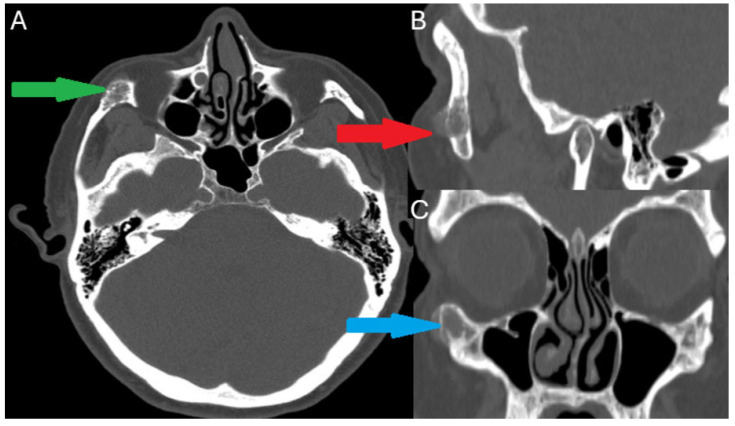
Because of atypical bone symptoms, more improved diagnostics were performed. Initial CT scan with contrast enhancement (GE Medical Systems, Optima CT 540, Bengaluru, India). In the axial view (green arrow), a radiolucent lesion of low density is seen within the body of the right zygomatic bone. The lesion has dimensions of 1.5 × 1.0 cm and a trabecular appearance (A). Within the lesion, radiopaque spikes are seen radiating outwards of the lesion following a sunburst pattern. On the lateral view, the red arrow points out that the lesion is well circumscribed, although the cortical layer of the zygomatic bone is eroded (B). There are no signs of periosteal reaction. Some atypical bone swelling and elevation with a mixed radiolucent-radiopaque lesion on the border of the inferior orbital rim and the zygomatico-maxillary junction is noted (blue arrow) on the coronal view (C). Similar radiologic findings in this area should be differentiated from fibrous dysplasia, osteochondroma, meningioma, osteogenic sarcoma, osteomyelitis, atypical bone inflammation, and multiple myeloma (MM). Based on the radiological findings, the suspicion of a benign intraosseous lesion was high. Because of the atypical location, symptoms, and radiological appearance, a decision for an excisional biopsy was made. We avoided the performance of a fine needle aspiration biopsy (FNAB), since the bone that covers the lesion makes it hard to penetrate, and the possibility of bleeding was high [1]. This decision was made because there were no worrisome symptoms like fatigue, weight loss, or anemia. On the other hand, because of the radiological appearance, authors initially differentiated the lesion between ossifying fibroma (OsF), CGCG (central giant cell granuloma), or an intraosseous hemangioma (IH). The suspicion for IH was high because of the characteristic pattern in CT scans. A well-circumscribed lesion with clear borders and a radiating trabecular appearance following a ‘’sunburst ‘’pattern can be pathognomonic for IH [2]. MRI can add valuable information regarding soft tissue involvement and is superior to a CT scan for the evaluation of vascular lesions [3]. Some studies reported that on MRI, IH are typically seen as hyperintense lesions on T2 images and hypointense or hyperintense on T1 images depending on the size of the lesion [3,4]. Generally, in radiological examination, after contrast, the lesion is more visible; however, small lesions are seen as hyperintense while larger lesions are hypointense on T1-weighted images [3,4]. The ratio of red and yellow (fatty) marrow within the lesion influences the MRI appearance of IH [2]. It is always worth estimating the periosteal condition and the status of adjacent bones. The absence of periosteal reaction made osteogenic sarcoma a less possible outcome than other entities. Intraosseous meningioma was also excluded because of the atypical site of that lesion and the absence of soft tissues. Fibrous dysplasia has a ground-glass appearance, and the lesion does not follow a radiating pattern. Osteochondroma was also excluded because of the irregular shape that it often has and the absence of corticomedullary continuity, a projection from the main bone which is characteristic of osteochondromas. Finally, there were no multiple lesions nor osteoporotic bone that would suggest multiple myeloma.
